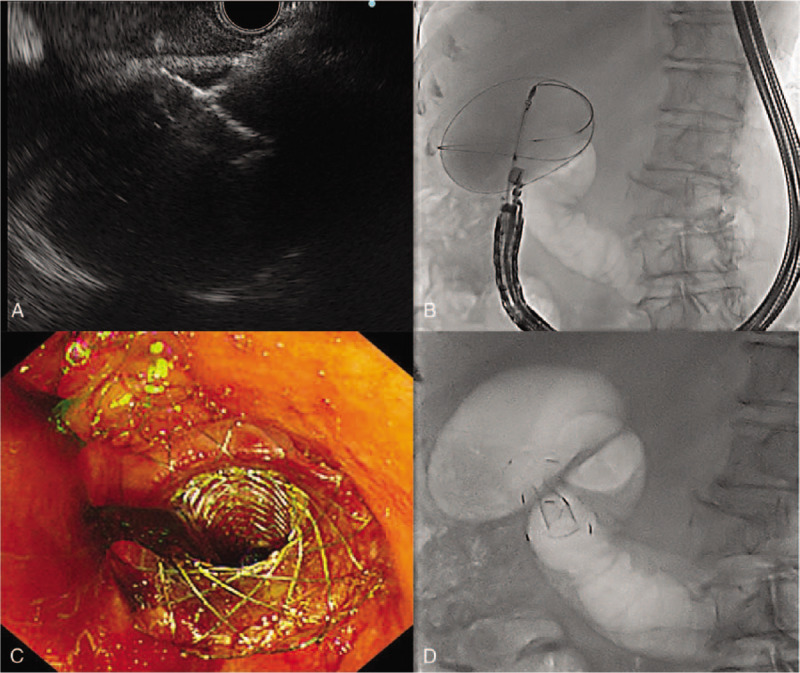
EUS-GBD using LAMS. (A) and (B) Puncturing GB and guidewire insertion. (C) and (D) After complete deployment of LAMS. EUS = endoscopic ultrasound; GBD = gallbladder drainage; LAMS = lumen-apposing metal stent.
